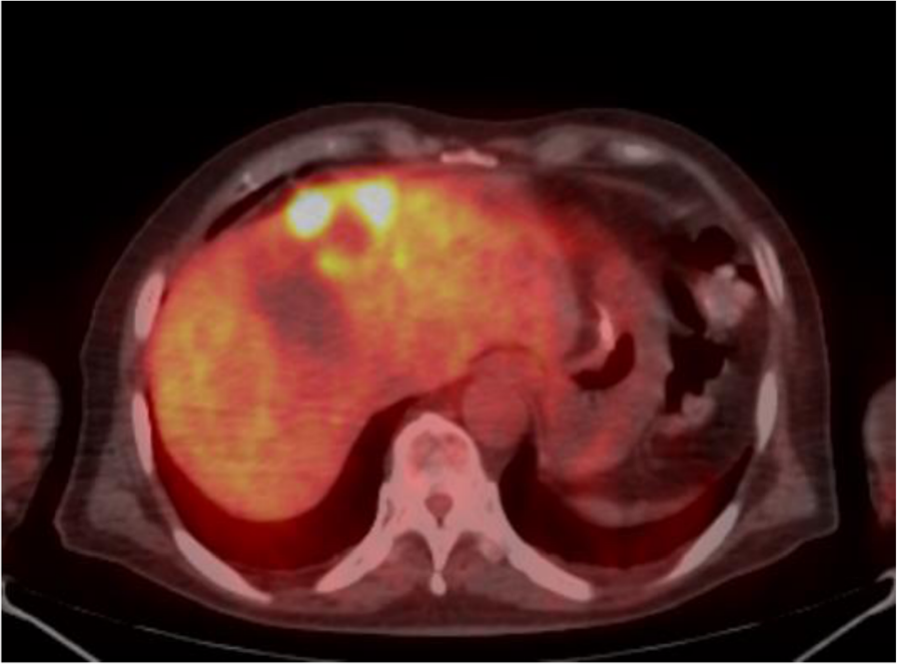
18F‑fluorodeoxyglucose positron-emission tomography findings. The S3+4 tumor verge represented high FDG accumulation (SUVmax=12.13)
